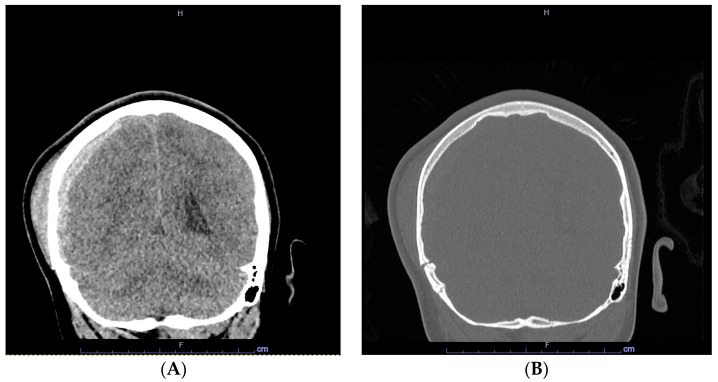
Head CT images of right acute subdural hematoma (A), and bone window of skull fractures (B).
